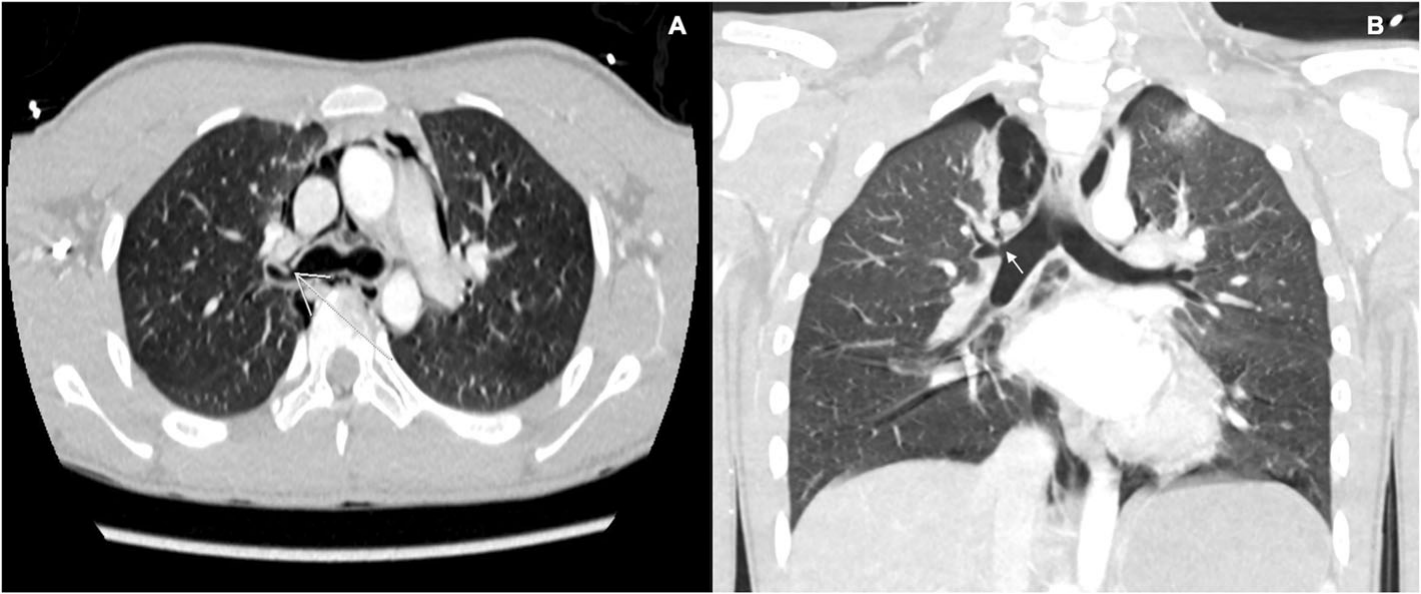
Computed tomography angiography. a Transverse section. Right main bronchus rupture and mediastinal emphysema. b Sagittal section. Right main bronchus rupture, mediastinal emphysema, and right pneumothorax
